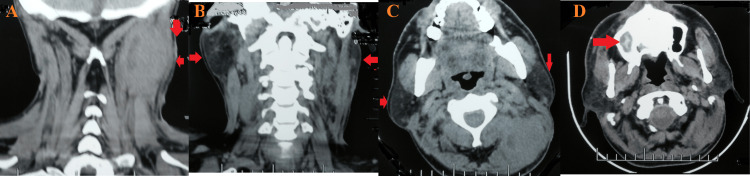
A plain CT of the neck CT: Computed tomography A: Left cervical lymph node involvement B: Bilateral cervical lymph node involvement C: Bilateral cervical lymph node involvement D: Right maxillary sinus involvement
